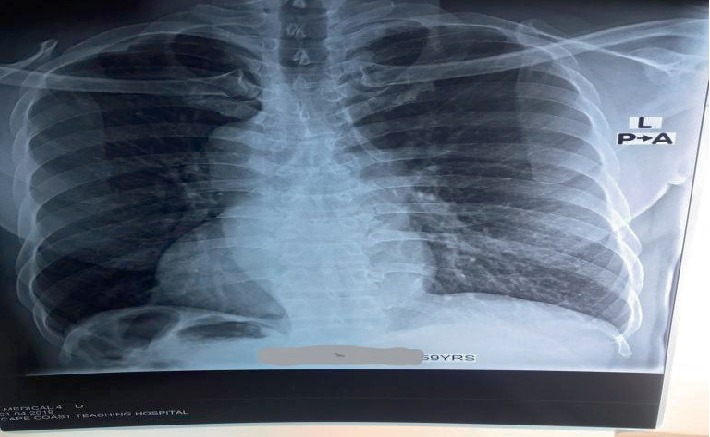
Frontal chest radiograph. It shows the apex of the heart pointing to the right, ascending and arch of the aorta on the right, gastric air bubble and splenic flexure both on the right side under the diaphragm opposite of the normal anatomical findings.
